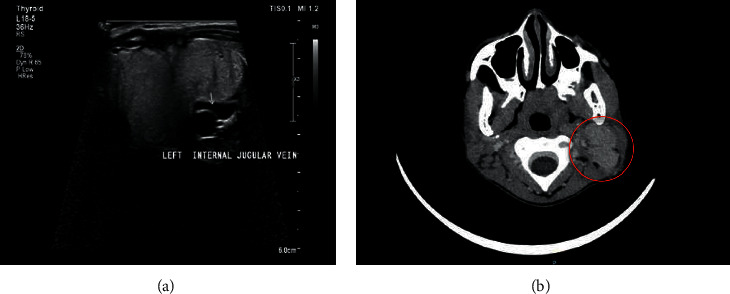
(a) Neck ultrasound shows enlarged left cervical lymph node compressing on the left internal jugular vein; (b) CT scan shows mattered left cervical lymphadenopathy (
